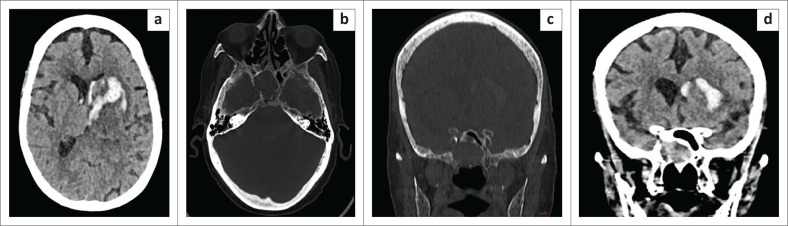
(a) Axial CT reveals intraparenchymal haemorrhage in the left gangliocapsular region with intraventricular extension. (b and c) Axial and Coronal CT demonstrates the defect in the right lateral wall of sphenoid sinus. (d) Herniated brain tissue within the sphenoid.
